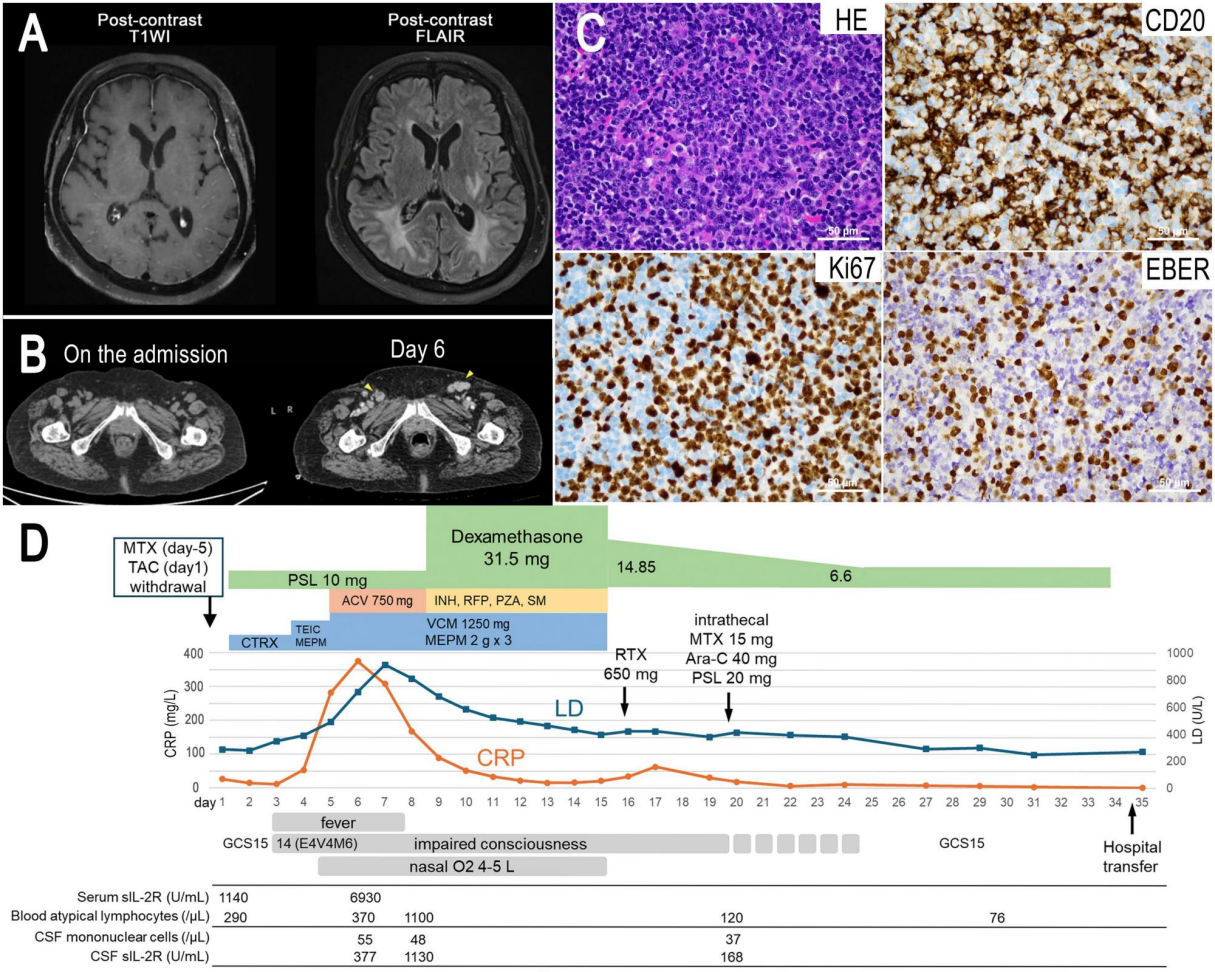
Imaging and clinical timeline. (A) The contrast-enhanced T1-weighted image (T1WI) on the left shows dural enhancement, and the contrast-enhanced fluid-attenuated inversion recovery (FLAIR) image on the right exhibits high signal intensities along the cerebral sulci. (B) Computed tomography (CT) images on admission and 6 days after admission. Yellow arrows indicate enlarged inguinal lymph nodes. (C) The inguinal lymph node biopsy showed a background of small lymphocytes with diffuse proliferation of large lymphocytes, consistent with EBER-positive diffuse large B-cell lymphoma (DLBCL). (D) Clinical timeline. Abbreviations: methotrexate (MTX); tacrolimus (TAC); teicoplanin (TEIC); meropenem (MEPM); vancomycin (VCM); acyclovir (ACV); isoniazid (INH); rifampicin (RFP); pyrazinamide (PZA); streptomycin (SM); rituximab (RTX); cytarabine (Ara-C); Glasgow Coma Scale (GCS); cerebrospinal fluid (CSF); soluble IL-2 receptor (sIL-2R)
